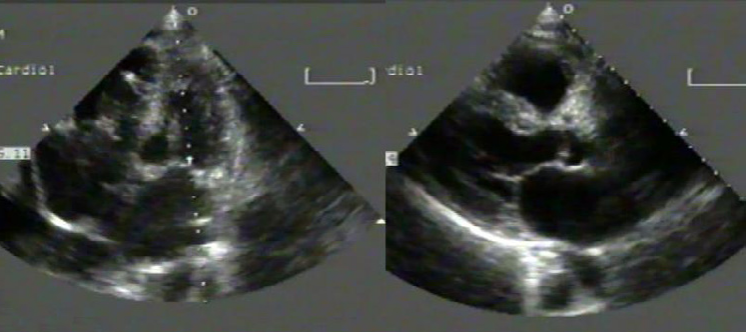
Two-dimensional transthoracic echocardiogram recorded in the marginal recipient with cardiac amyloid. In both the parasternal long-axis (right) and apical four-chamber (left) images note the homogeneous echo intensity of the myocardium, which is noted as a bright "speckling" appearance. Both the mitral and aortic valves are also thickened. The patient had severe ventricular dysfunction and advanced heart failure symptoms (NYHA Class IV). (Images from Marinella Ferlito, Director Echo lab, and Prof Claudio Rapezzi, University Cardiology Division, S. Orsola Hospital, Bologna, Italy) Additional files (video clips) 5 and 6. Two-dimensional transthoracic echocardiogram of the donor heart grafted in the marginal recipient. In both the parasternal long-axis (Additional file 5) and apical four-chamber (Additional file 6) views note the excellent global and regional ventricular function. Chambers and cardiac structures are normals. (Images from Marinella Ferlito, Director Echo lab, and Prof Claudio Rapezzi, University Cardiology Division, S. Orsola Hospital, Bologna, Italy)
